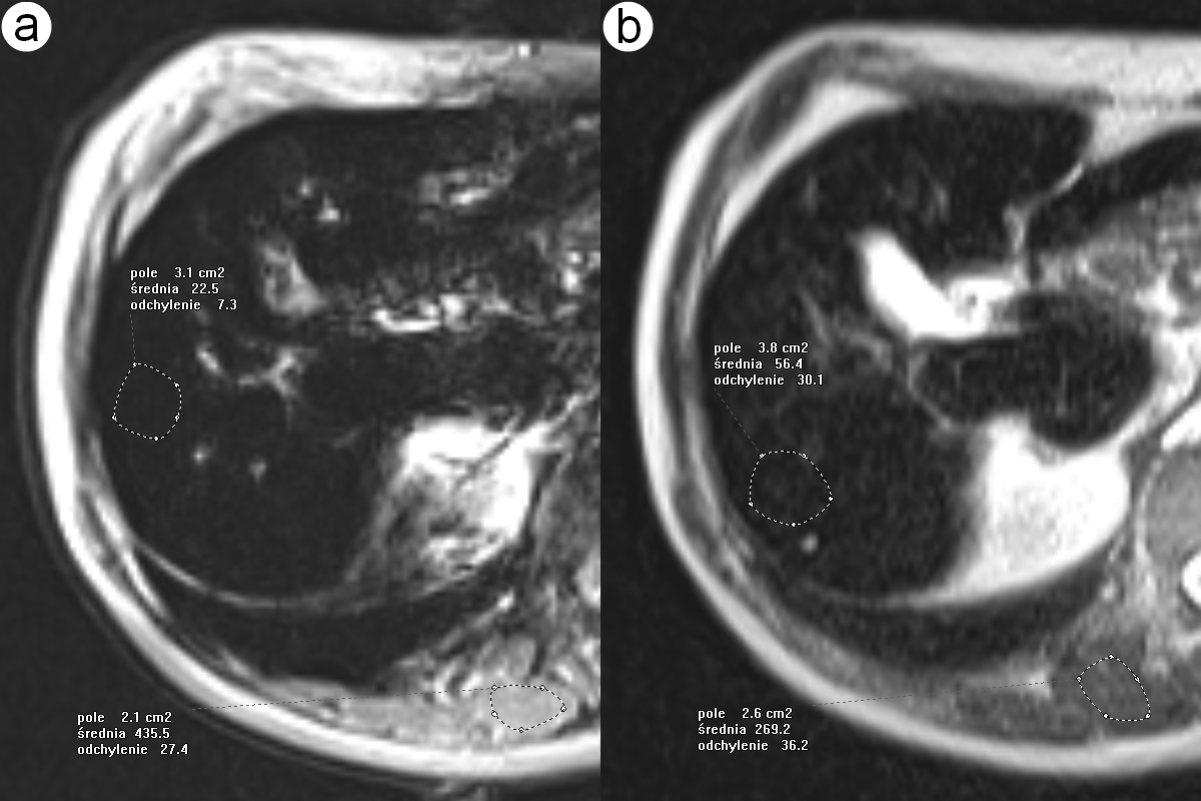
68-year-old man with cirrhosis and hemosiderosis confirmed by the biopsy. Axial MR T2-weighted images performed on gradient echo (fig.2a) and Express (fig.2b) sequences show typical findings for hepatic iron overload. The signal intensity of liver (accordingly 23 and 56) is lower than that of paraspinal muscles (accordingly 436 and 269).
